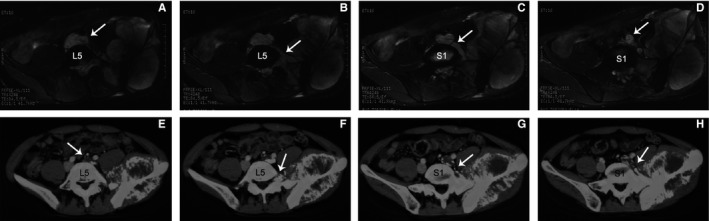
Typical cases of pelvic osteosarcoma with venous tumor thrombus (VTT) and involvement of the L5/S1 intervertebral foramen. A‐D, A 21‐year‐old male diagnosed as a type I+II+III+IV pelvic osteosarcoma of chondroblastic subtype. A, He was complicated by a VTT extending from left internal iliac vein to the left common iliac vein and the inferior vena cava (arrow). B, Axial MRI scans showed obvious involvement of the left L5/S1 intervertebral foramen (arrow). C‐D, A branch of the internal iliac vein filled with tumor thrombus surrounded the S1 vertebra linking the tumor with the common iliac vein (arrow). E‐H, A 26‐year‐old male diagnosed as a type I+II+III+IV pelvic osteosarcoma not otherwise specified. E, He was complicated by a VTT extending from left internal iliac vein to the left common iliac vein (arrow). F, Axial CT scans showed obvious involvement of the left L5/S1 intervertebral foramen (arrow). G‐H, A branch of the internal iliac vein filled with tumor thrombus surrounded the S1 vertebra linking the tumor with the common iliac vein (arrow)
